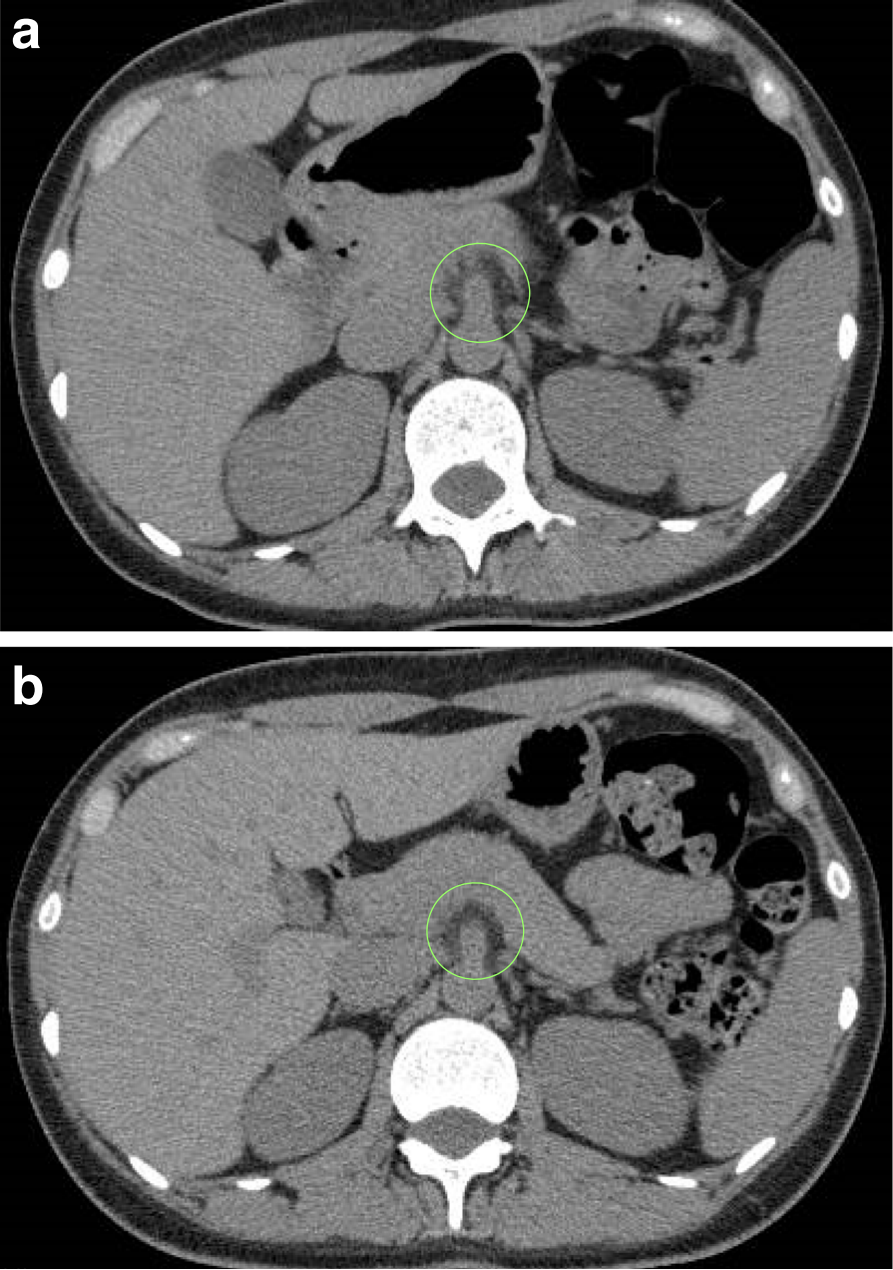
a Abdominal computed tomography (CT) findings on Day 79: Mild increases in CT values were seen around the celiac artery and the root of the superior mesenteric artery. b Abdominal CT on Day 104: The findings compared with those of Day 79 have changed to approach normal levels
